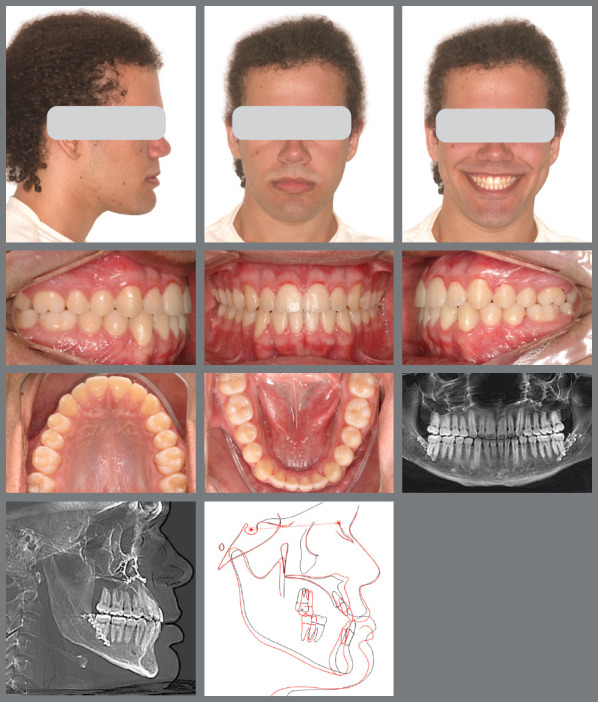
Facial and intraoral images and radiographs at the final post-treatment stage.
